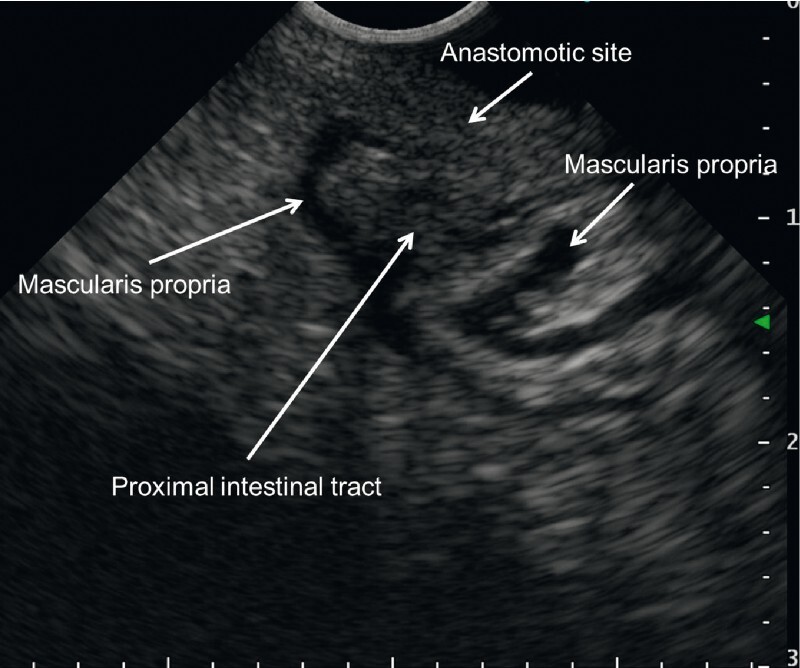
Gel immersion endoscopic ultrasound view clearly showed the proximal intestinal tract.
